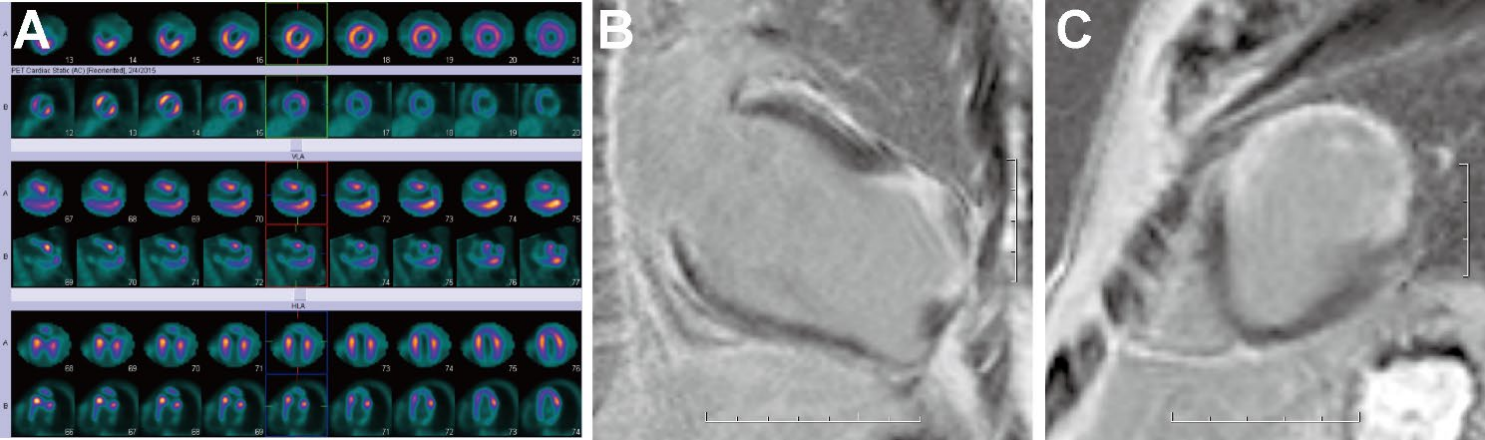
Single-Photon Emission Computed Tomography (SPECT), 18 F-fluorodeoxyglucose (18 F-FDG) myocardial metabolic imaging, and magnetic resonance imaging (MRI). (A). SPECT and 18 F-FDG myocardial metabolic imaging demonstrated impaired blood perfusion and metabolism in the apex and part of apical segments of anterior wall of the left ventricle. (B), (C). The myocardial delayed-enhanced scan showed transmural late gadolinium enhancement (LGE) of the thinned area above
